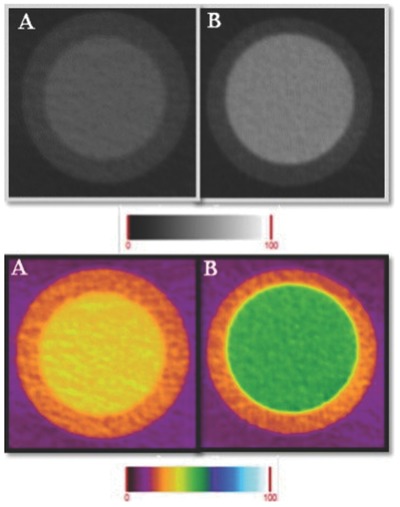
Micro‐CT grayscale (upper panel) and colored (lower panel) images of A) water and B) PEGylated Au NPs. Adapted with permission.^66^ Copyright 2015, Elsevier.
